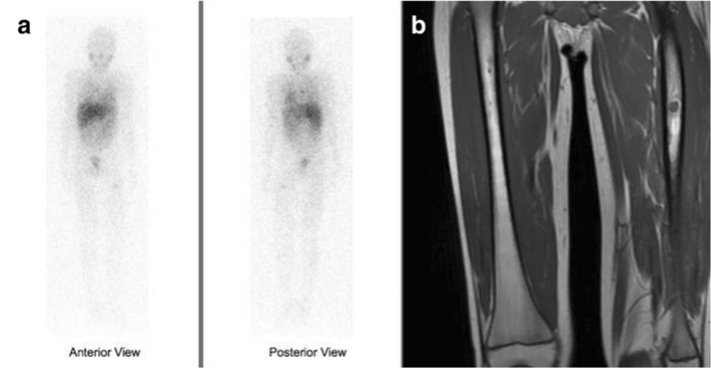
Scanning with 123-metaiodobenzylguanidine (I-123-MIBG) showing uptake on the proximal left femoral bone, suggestive of metastatic disease (a). Lower limb MRI revealing a proximal femoral lesion (b)
